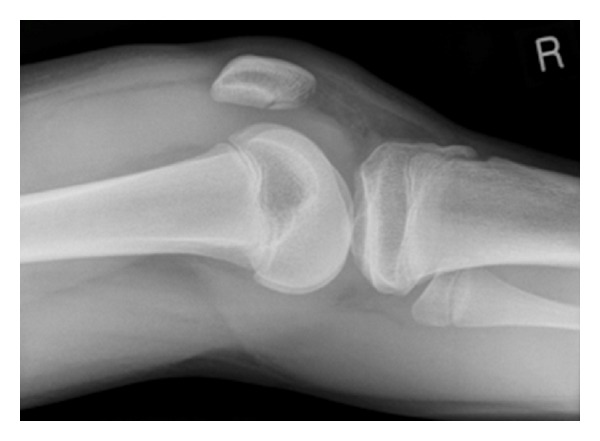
Lateral radiograph of the right knee taken on initial presentation.
